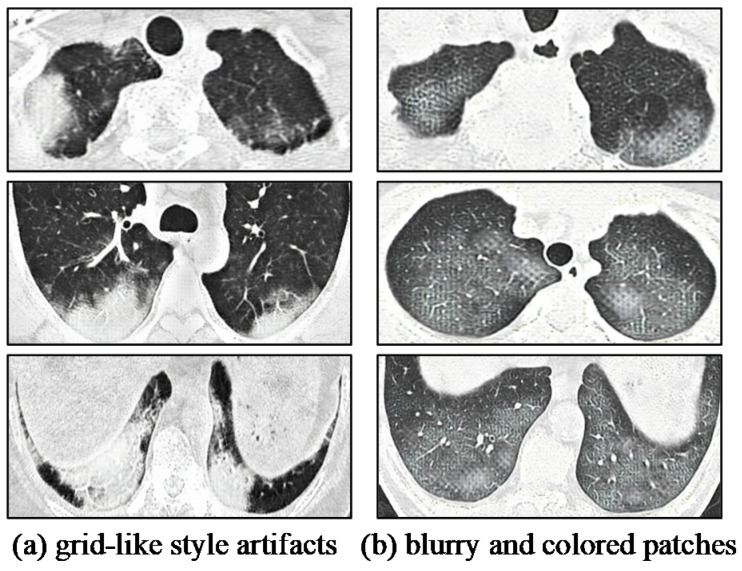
(a,b) Examples of style-artifacts manifested on synthetic COVID-19 CT images. These samples are generated from other GAN baselines.
